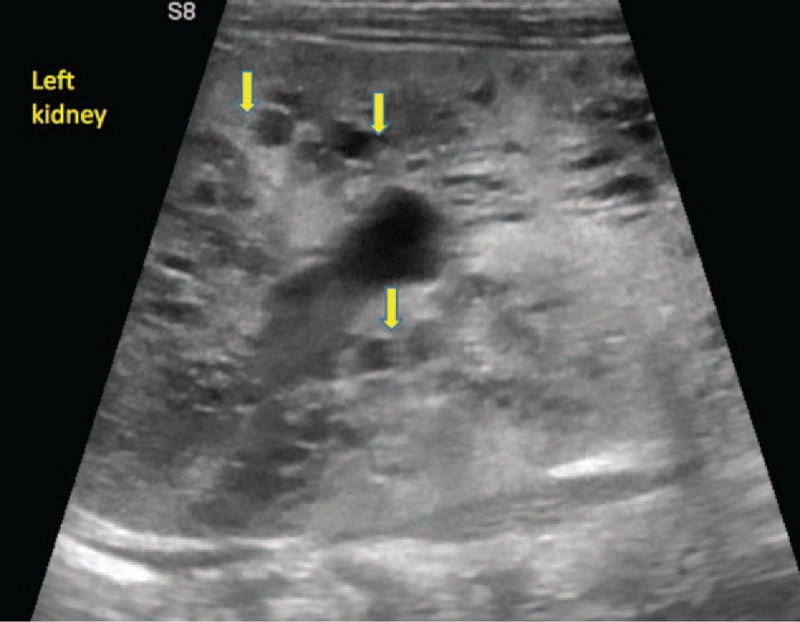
The ultrasound aspect of cystic lesions in the left kidney at the time of diagnosis.
